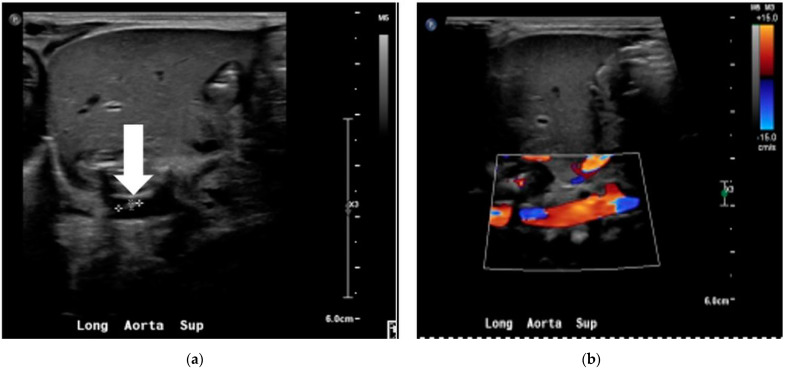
Aortic doppler study showing the non-occlusive thrombus in the grayscale (a) and color doppler (b).
